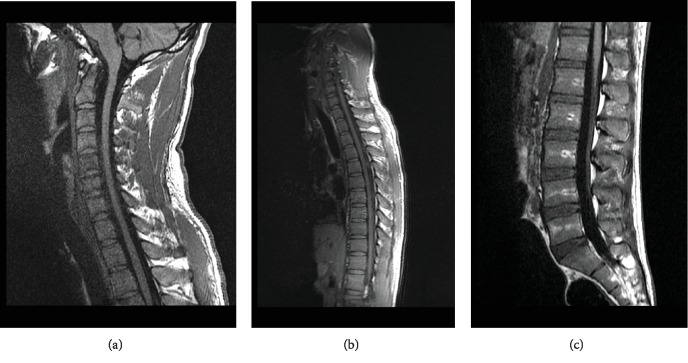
MRI of the cervical (a), thoracic (b), and lumbar spine (c) showing no evidence of leptomeningeal spread.
